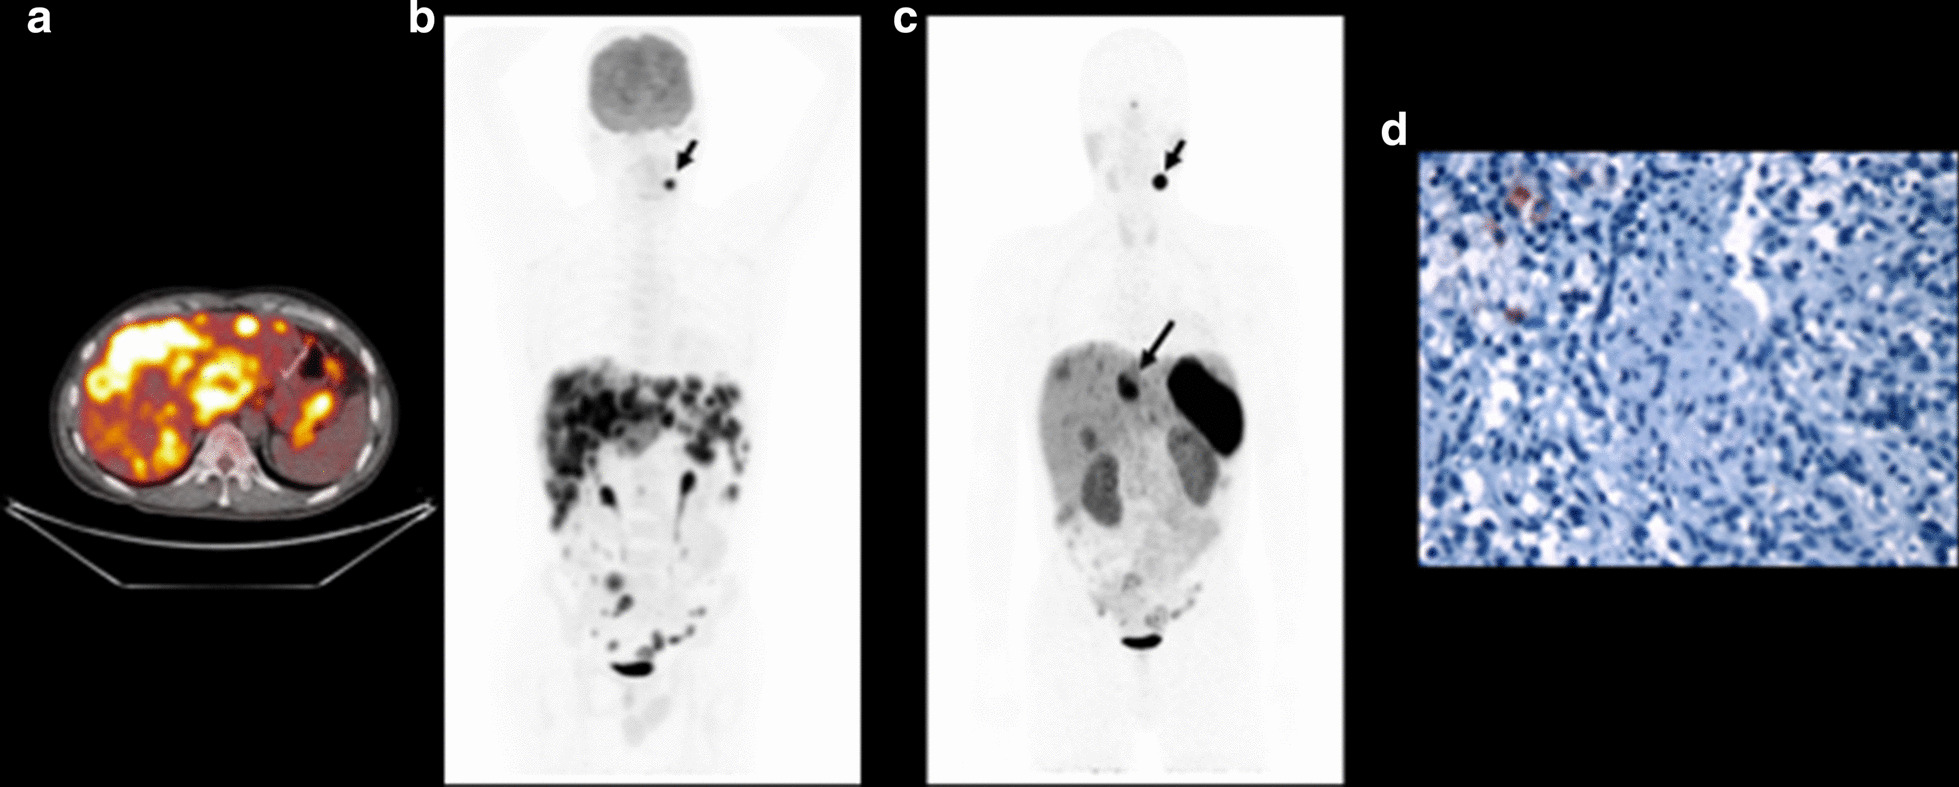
Case 002. Panel A, transaxial fused [^18^F]FDG PET/CT image of the liver and MIP image demonstrating FDG-avid liver, peritoneal and nodal metastases as well as a synchronous left sided carotid paraganglioma (small arrow). Panel B, [^68^ Ga]Ga-DOTATATE PET/CT MIP image, demonstrating that few liver metastases show uptake higher than background liver (SUVmax 13, large arrow, vs 6.5 for background liver). It should be noted that not all liver lesions were biopsied so it remains unclear if all liver lesions were metastatic wtGIST deposits or if the carotid paraganglioma was also metastatic to the liver. The left sided carotid paraganglioma shows very intense uptake of the somatostatin analogue (SUVmax 60, small arrow). Panel C, negative SSTR2 expression on IHC in a biopsied dSDH wtGIST liver metastasis from case 002
